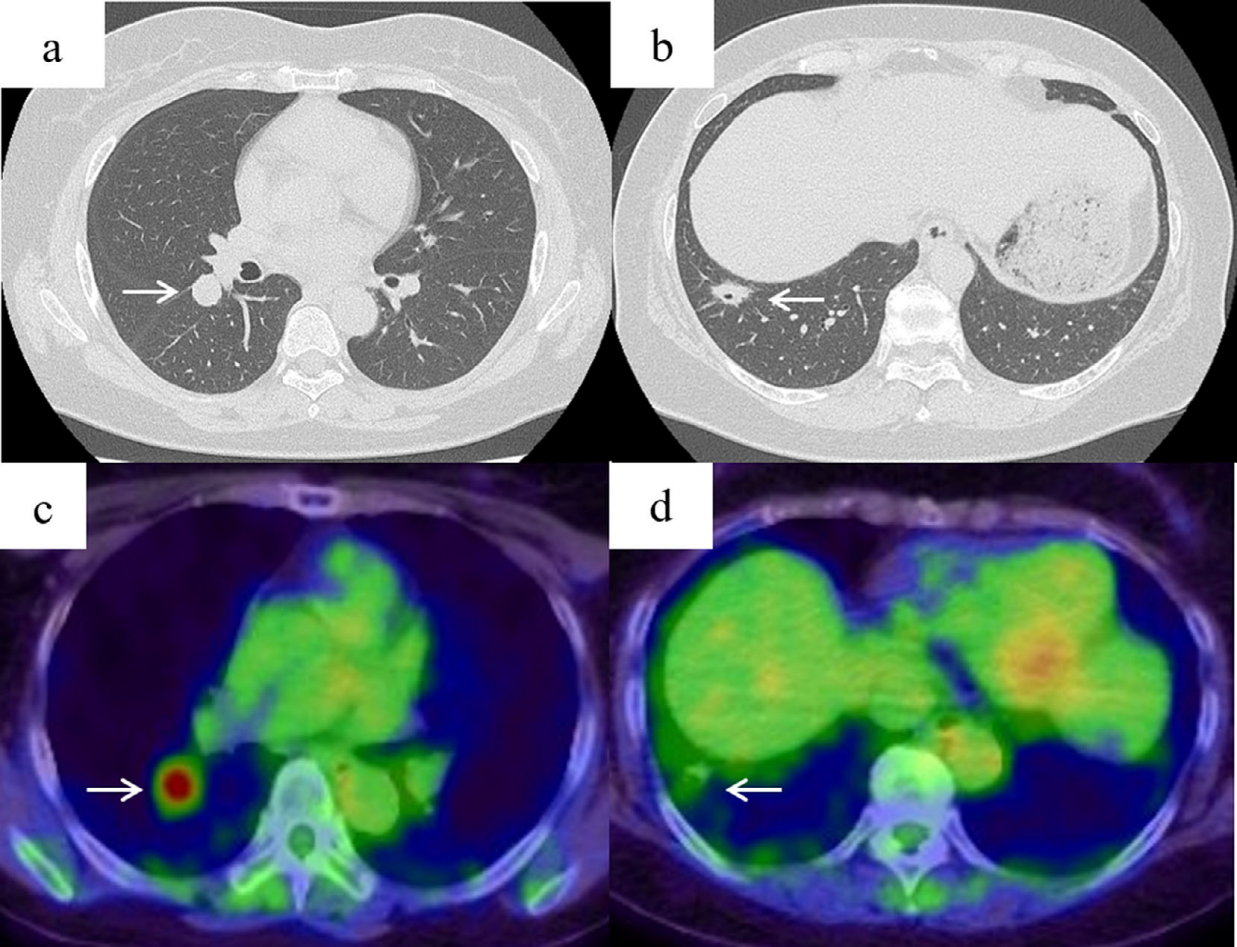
Chest computed tomography (CT) showed two nodules in the right lower lobe (arrow). (a) A well defined nodule; and (b) an irregularly‐defined nodule on air bronchograms. Both nodules exhibited tracer uptake (arrow) during 2‐[^18^F]‐fluoro‐2‐deoxyglucose positron emission tomography‐computed tomography, with (c) a maximum standardized uptake value (SUVmax) of 6.6; and (d) a SUVmax value of 2.0.
